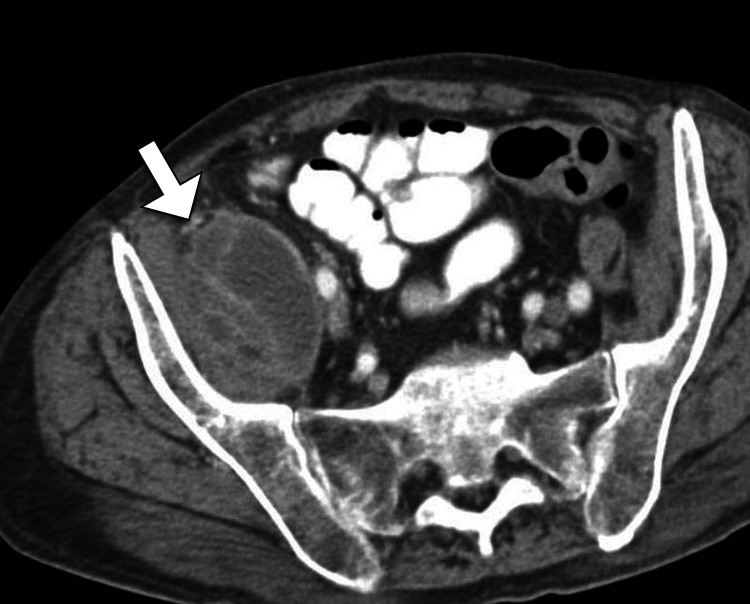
Axial CT image of the pelvis reveals a collection within the right psoas muscle (arrow) with a thickened enhancing wall, consistent with an abscess CT: computed tomography
